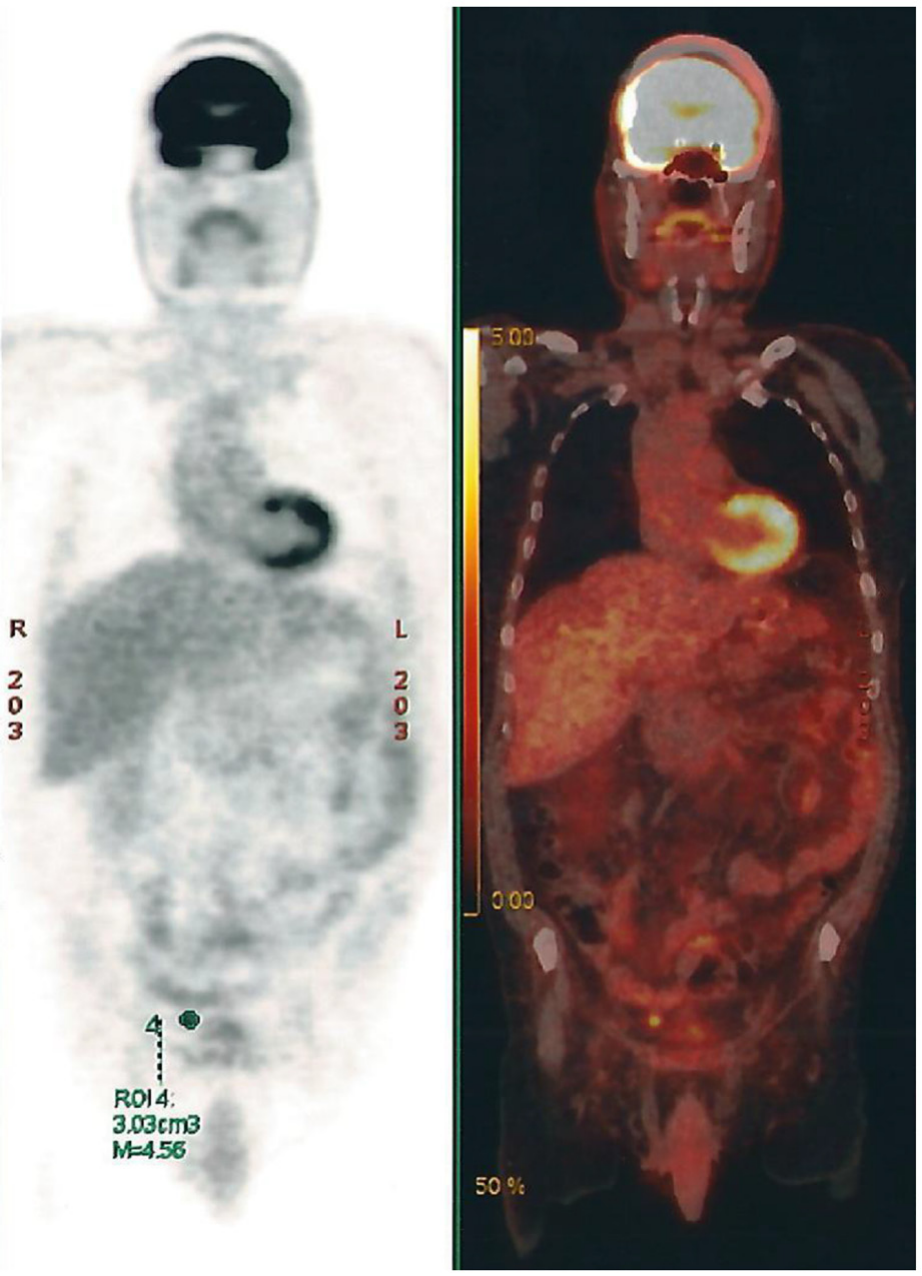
PET-CT Scan showing multiple retroperitoneal, pelvic and abdominal lymph nodes.
